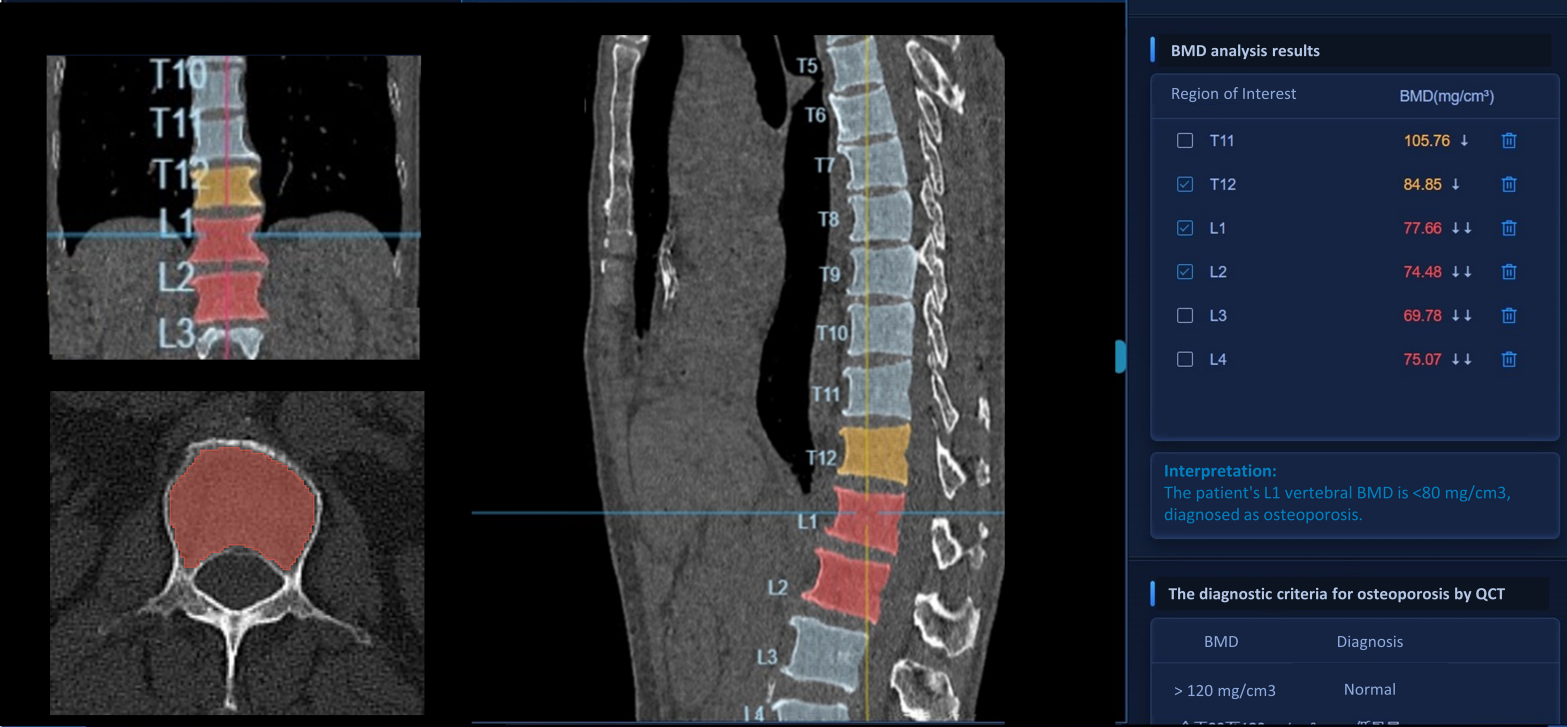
The detailed AI interface of BMD measurement. The left column of this interface comprises, from top to bottom, the patient’s coronal, axial, and three-dimensional reconstructed CT images, respectively. The middle column displays the patient’s sagittal CT images, while the right column presents the corresponding BMD results of the vertebrae. Vertebrae with BMD results <80 mg/m3 are annotated in red on the CT images, along with their respective BMD values. Vertebrae with BMD results in the range of 80-120 mg/m3 are marked in yellow on the CT images, together with their corresponding BMD values. BMD, bone mineral density; CT, computed tomography; AI, artificial intelligence.
